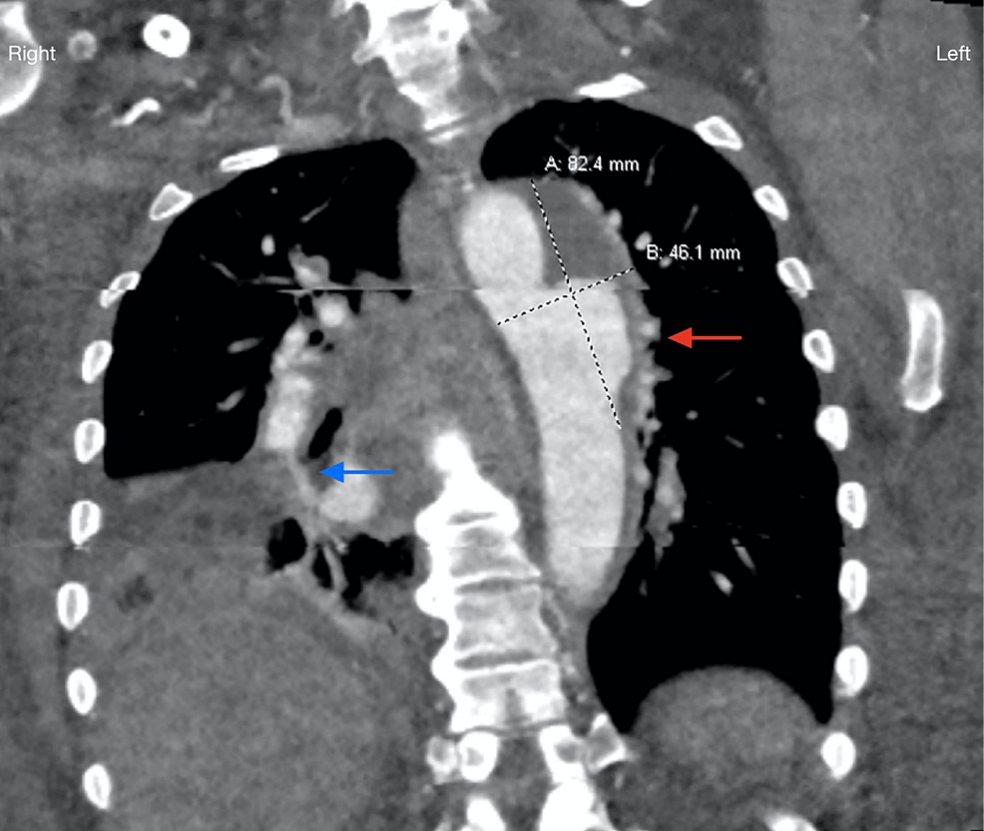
Computed tomography angiography of the chest showed an eccentric aneurysm involving the distal aortic arch and descending thoracic aorta partially thrombosed (red arrow). Multiple pulmonary emboli. Opacification in the right lung may reflect early infarction (blue arrow).
